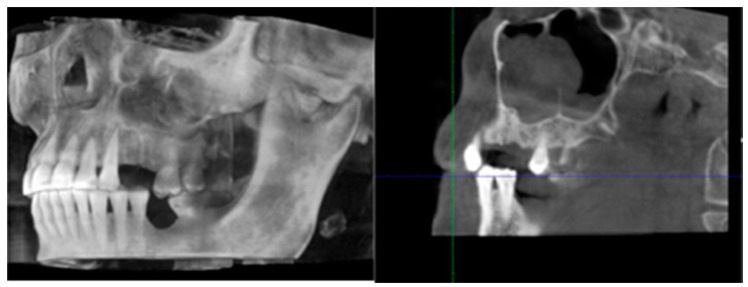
MRONJ “ghost socket” of the upper jaw as shown in 3D rendering, and coronal view of a cone beam computed tomography (CBCT) scan.
